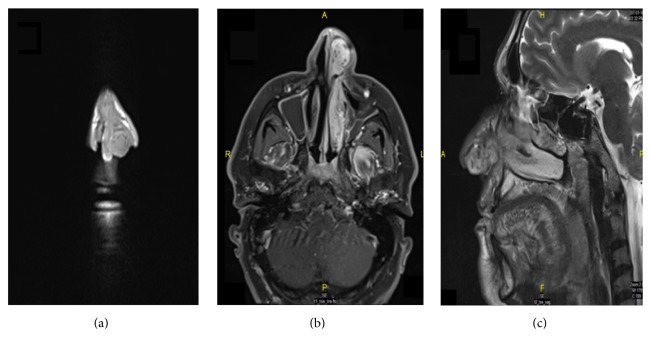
MRI of nose and paranasal sinuses showing a highly vascular mass occupying the left nasal vestibular area. (a) shows T1 coronal view, (b) shows T1 postcontrast axial view, and (c) shows a T2 sagittal view.
